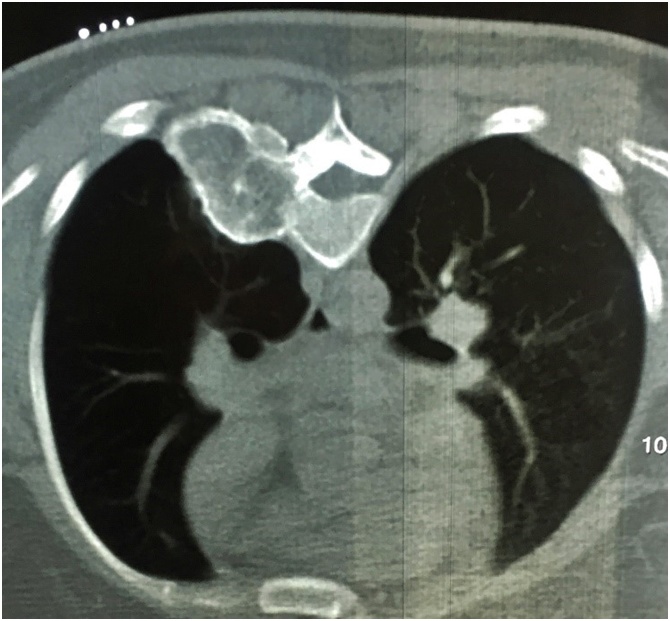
Chest Computed Tomography scan revealing the focal hematopoietic hyperplasia at the 6th rib.
